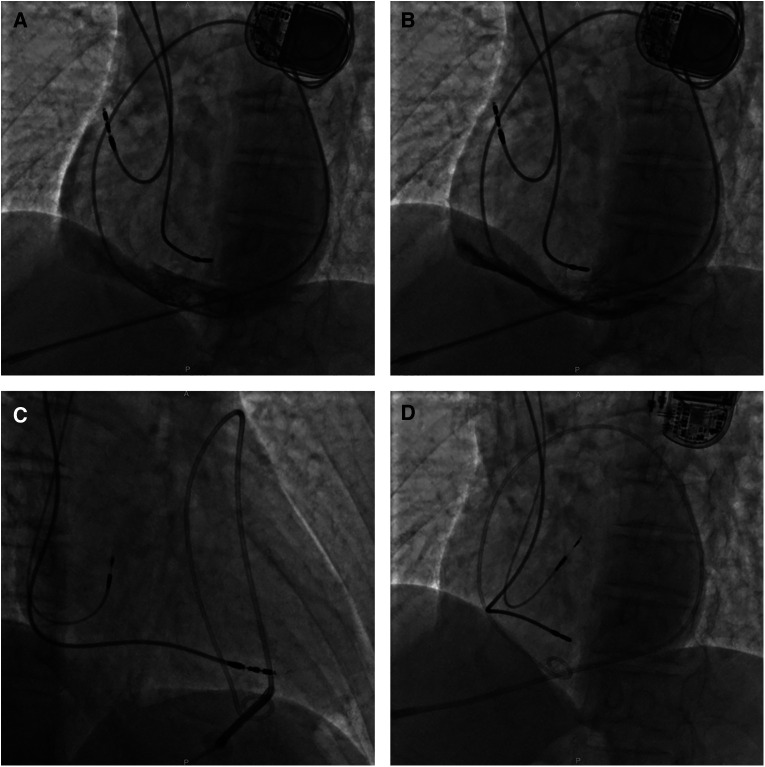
A fluoroscopy image of case 3 showing initial active fixation lead in lateral RA and moderate pericardial effusion [(A) LAO view]. X-ray guided pericardiocentesis was performed using an LAO view and a pigtail catheter was inserted into the epidural space for continuous drainage, thus hemodynamic stability was obtained (B). Then a new active fixation electrode was implanted in RA septum [(C) RAO view and (D) LAO view]. LAO, left anterior oblique; RA, right atrium; RAO, right anterior oblique.
